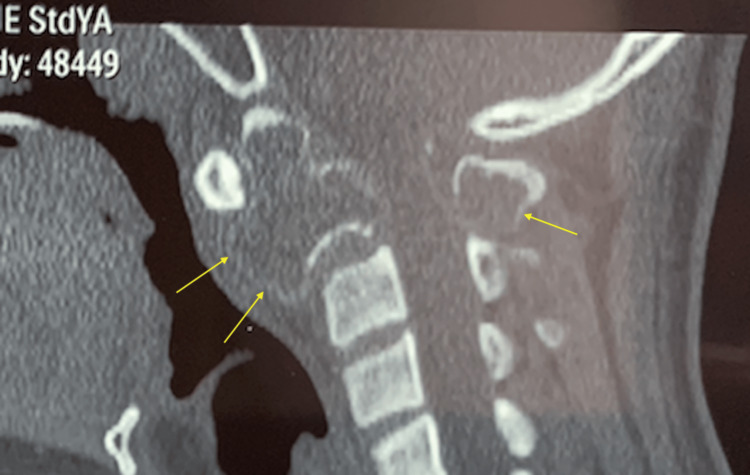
Sagittal CT of the upper cervical spine Yellow arrows show the cortical border of the C2 vertebra which has expanded and thinned out. The lesion has affected the anterior and posterior columns of the vertebra involved.
